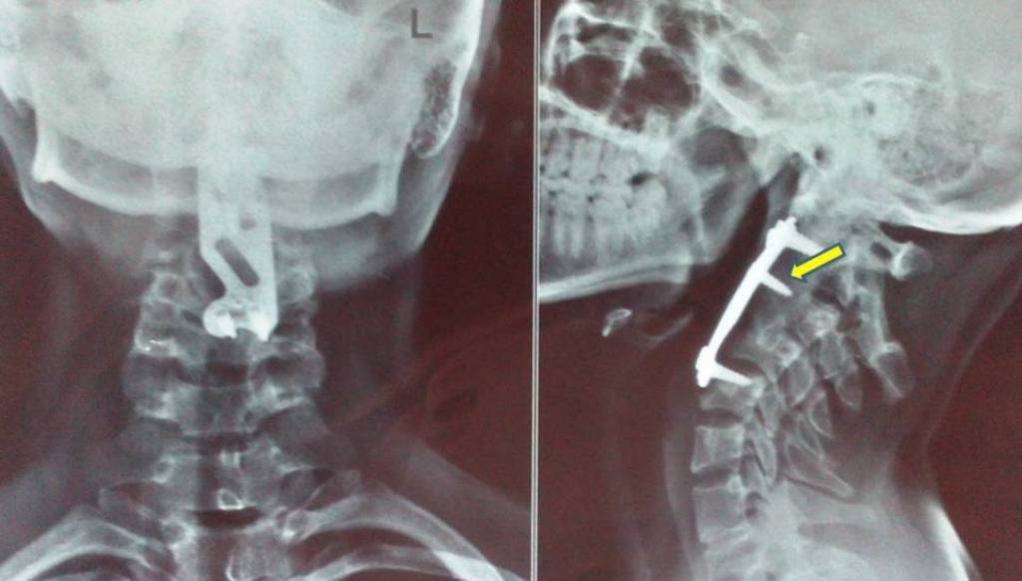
Follow-Up X-Rays, Anteroposterior View (left) and Lateral View (right) of the Cervical Spine Good incorporation of the tricortical iliac crest graft (arrow).
